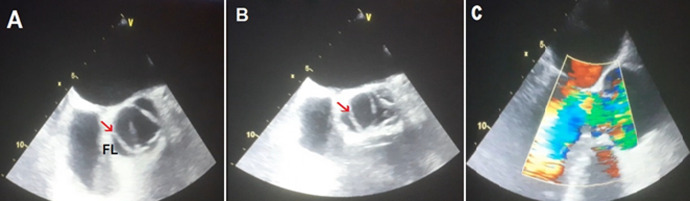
transesophageal echocardiogram: (A) short axis view of the aortic valve in systole showing the dissection flap (arrow) and right coronary artery arising from the false lumen of dissection (FL); (B) in diastole showing the incomplete closure of the aortic valve; (C) long axis view with color doppler demonstrating severe aortic regurgitation
